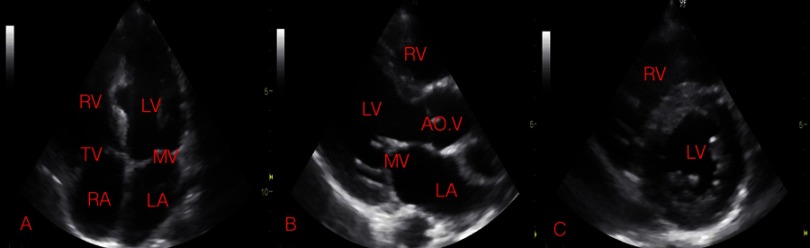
Echocardiographic frames taken 3-years after removal of hydatid cyst from the interventricular septum (A. 4-chamber view, B. Parasternal long axis view, C. Parasternal short axis view), with the only evident residual abnormality being a thinned area in the interventricular septum at the site of the prior cyst.
